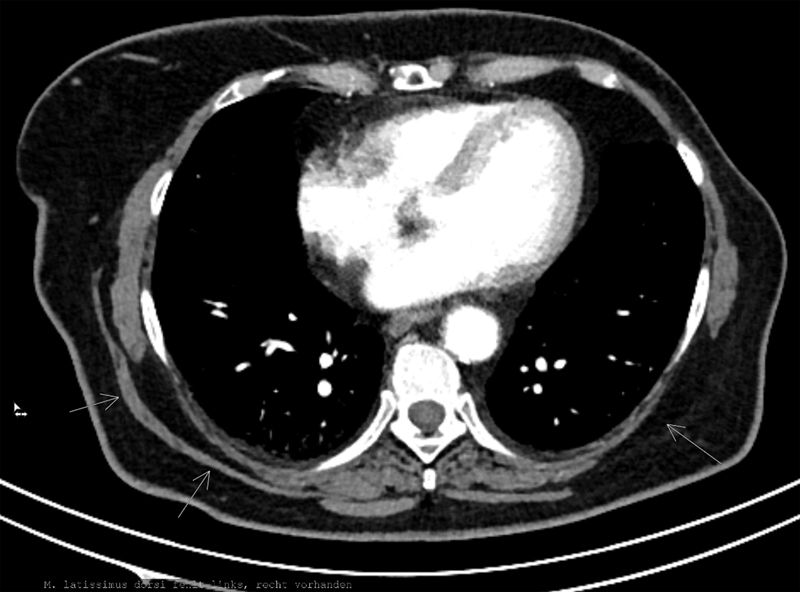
Preoperative (2 years before) CT scan, showing the unilateral latissimus dorsi muscle absence. CT, computed tomography.
